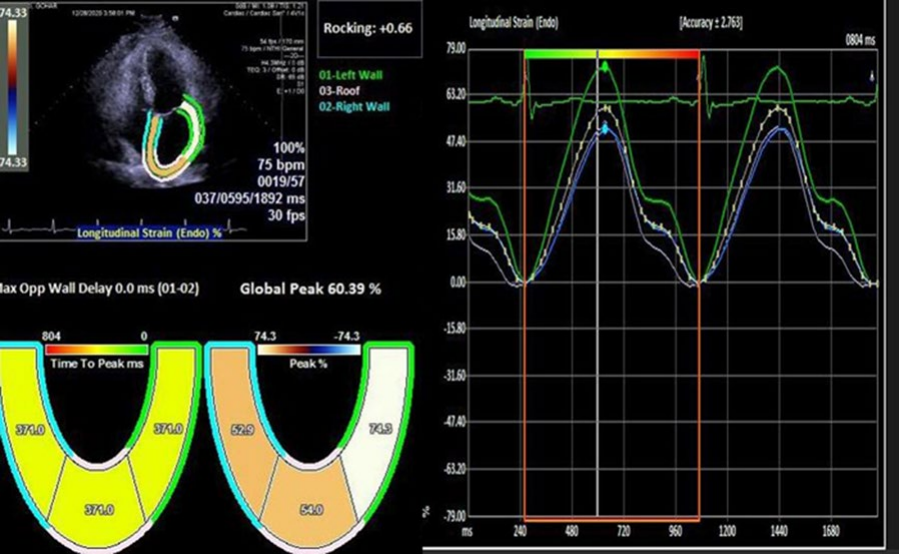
Speckle-tracking echocardiography in apical four chamber view represents longitudinal LA reservoir strain curves in three segments containing left LA wall, roof, and right LA wall by considering the QRS complex (R-R gating) as the initiation of the strain calculation (*LA* Left atrium)
